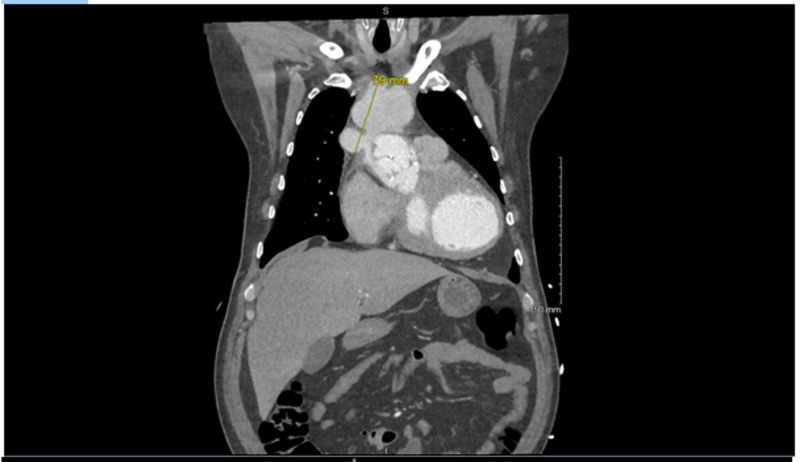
Coronal view of CT scan demonstrating the pseudoaneurysm of the aorta; yellow line demarcates the pseudoaneurysm with exact dimensions.
